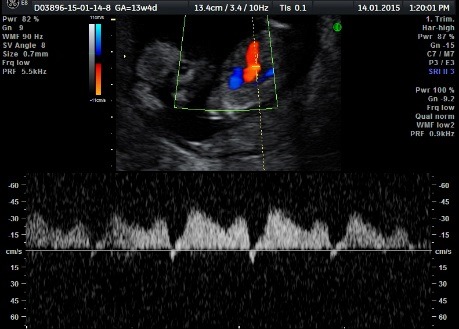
Doppler sonography of the developed normal fetus (pumping twin): ductus venosum having a negative “a” wave
